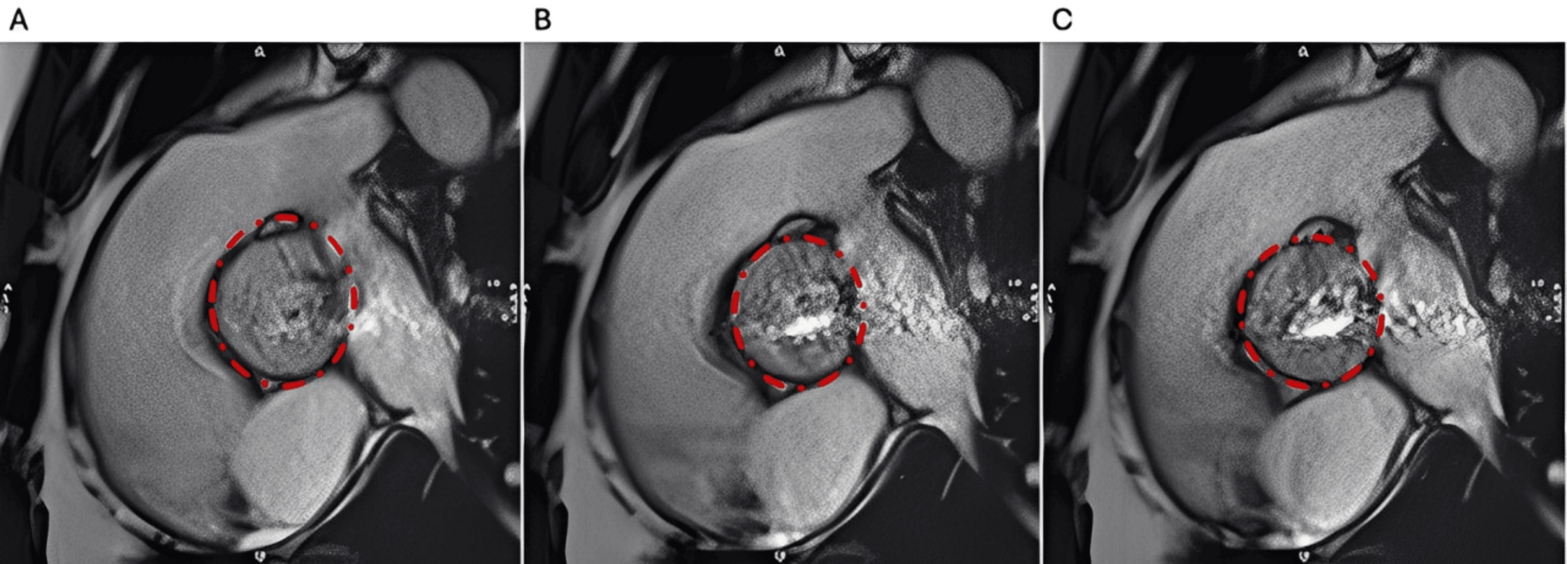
Cardiac MRI still frames of a UAV obtained with SSFP cine sequences during different phases of the cardiac cycle: (A) diastole, (B) mid-systole, and (C) end-systole The red dashed circle indicates the aortic valve. SSFP, steady-state free precession; UAV, unicuspid aortic valve
